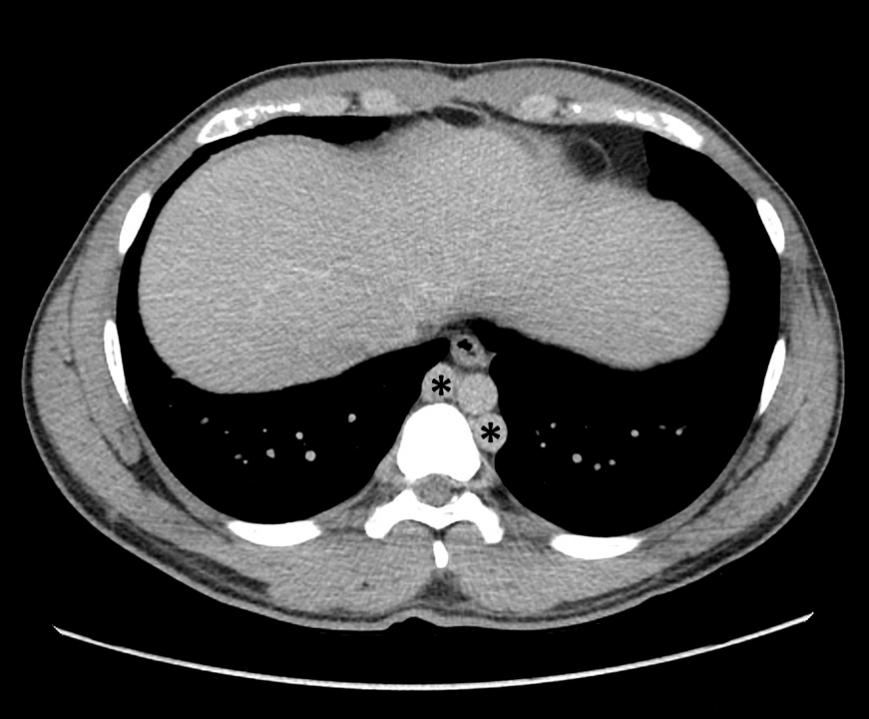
CT at the level of the lower chest, demonstrating dilation of both Azygos and Hemiazygos veins (asterisks), serving as the cephalad alternative venous drainage route due to the interrupted IVC. IVC, inferior vena cava.
